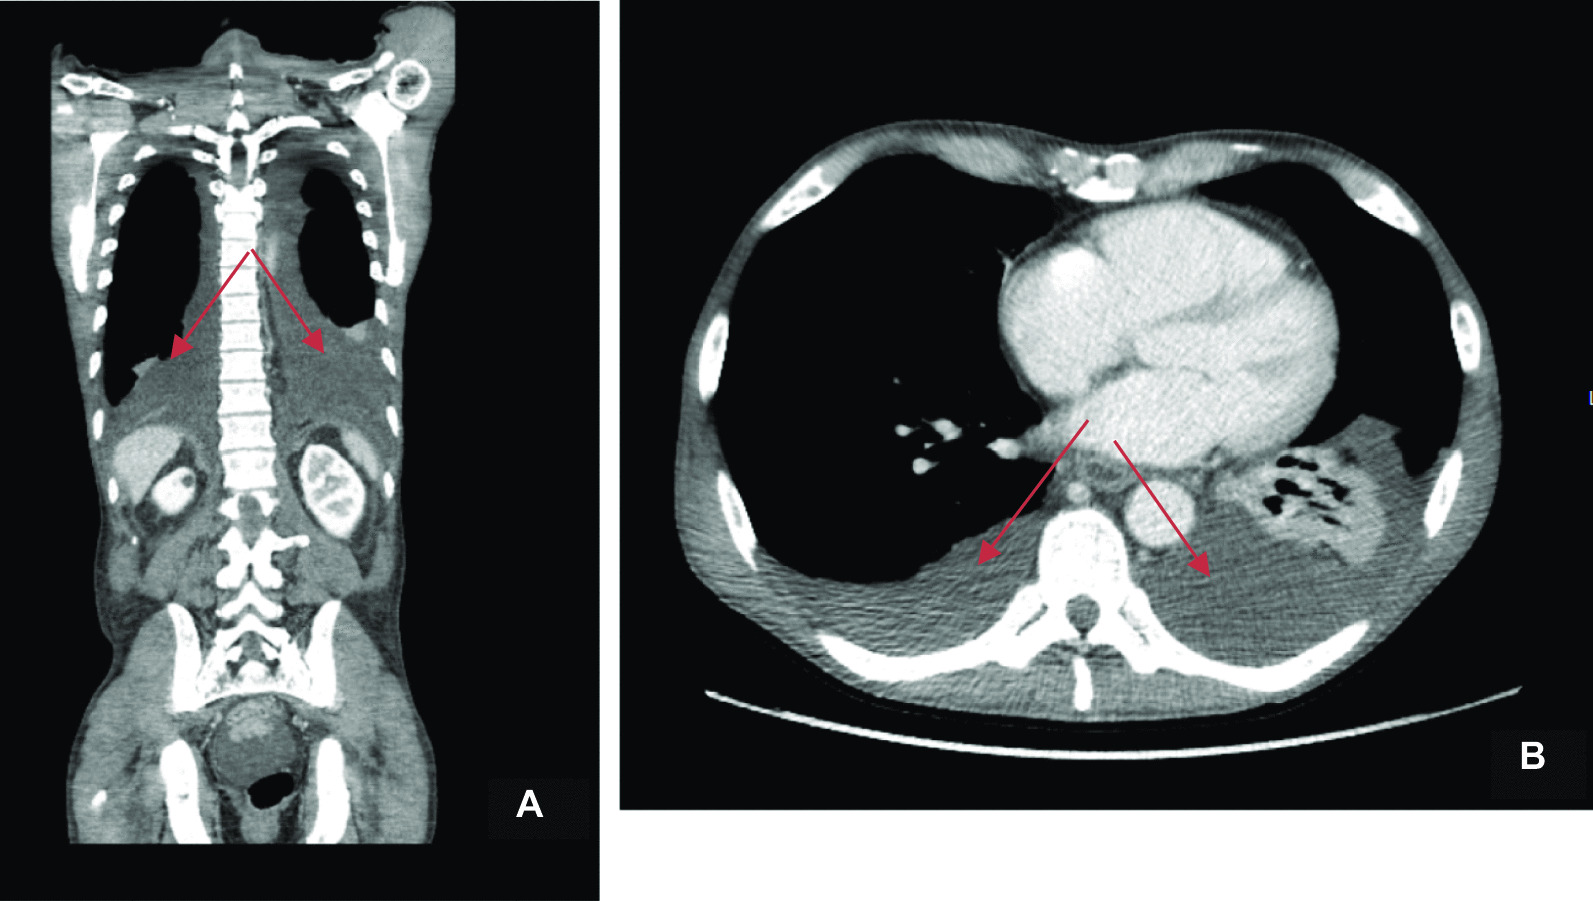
A, B CT chest–abdomen showing bilateral pleural effusion (indicated by arrows)
